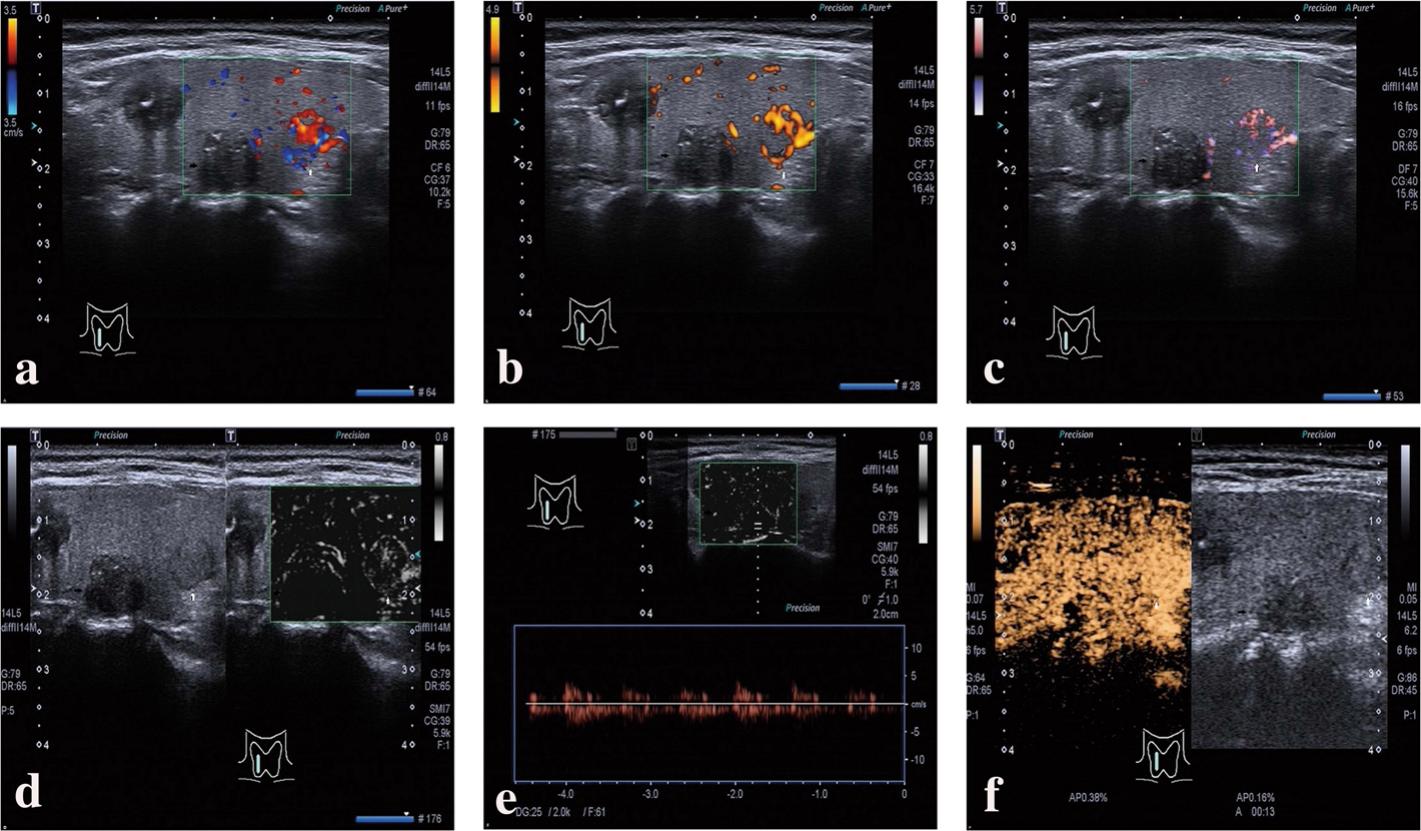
A papillary thyroid carcinoma (➔) and an adenoma (⇑) coexist in the right lobe. a CDI and b PDI show peripheral vascularization and peripheral interrupted vascularization of the nodule and internal microvascularization. c Color SMI and d monochrome SMI show more vascularization, in particular curved and ring peripheral vascularization surrounding the nodule as well as internal microvascularization with small branching details. e Pulsed Doppler (guided by monochrome SMI) established that the small branching microvasculature was real and measurable. f CEUS shows disordered heterogeneous hypoechoic enhancement with incomplete peripheral enhancement (➔) and ring enhancement around the nodule and homogeneous hyperechoic internal enhancement (⇑).
